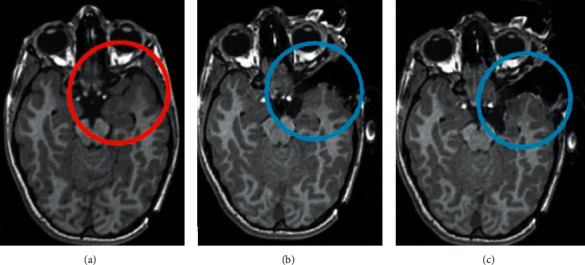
MRI of a patient with glioma before (a), during (b), and after (c) resection.
